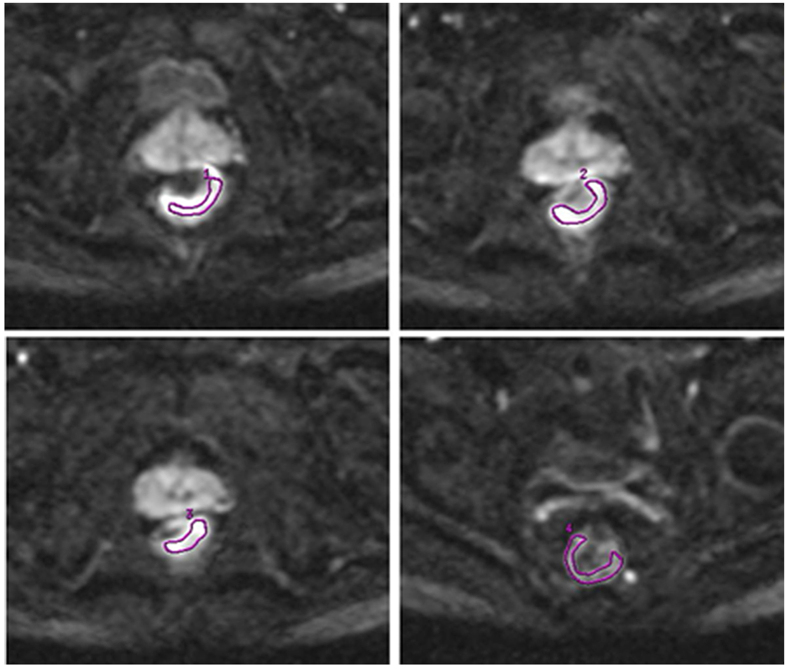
ROIs (1–3) were drawn on three consecutive slices with three of the largest cross-sectional areas of rectal tumor. The final value of the tumor’s ROI was the average of ROIs (1–3). ROI 4 (normal rectum) was drawn on an adjacent slice to the tumor. All ROIs were manually drawn on the DWI image with b = 1000 s/mm^2^ and the area of each ROI was not less than 100 mm^2^.
